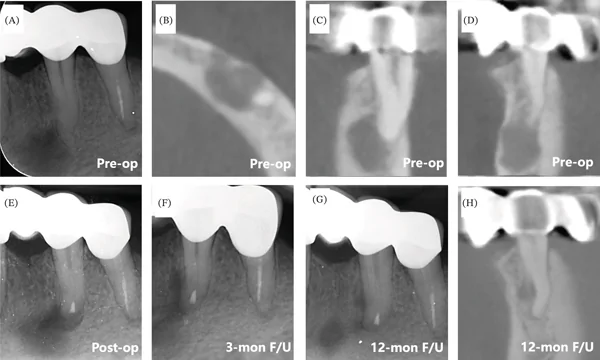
Preoperative and postoperative imaging of Case 3 (Tooth #21). (A) Preoperative PA demonstrating root curvature and a mesial radiolucency associated with Tooth #21. (B–D) Preoperative CBCT images revealing complete radiographic obliteration of the middle and apical root canal, an extensive mesial radiolucent lesion, and severe resorption of the buccal cortical plate of Tooth #21. (E) Immediate postoperative PA of Tooth #21. (F) Three‐month follow‐up radiograph depicting significant resolution of the periapical radiolucency. (G,H) Twelve‐month follow‐up PA and CBCT image confirming complete healing of the periapical lesion, with only a small residual radiolucency remaining on the mesial aspect. CBCT, cone beam computed tomography; PA, periapical radiograph.
